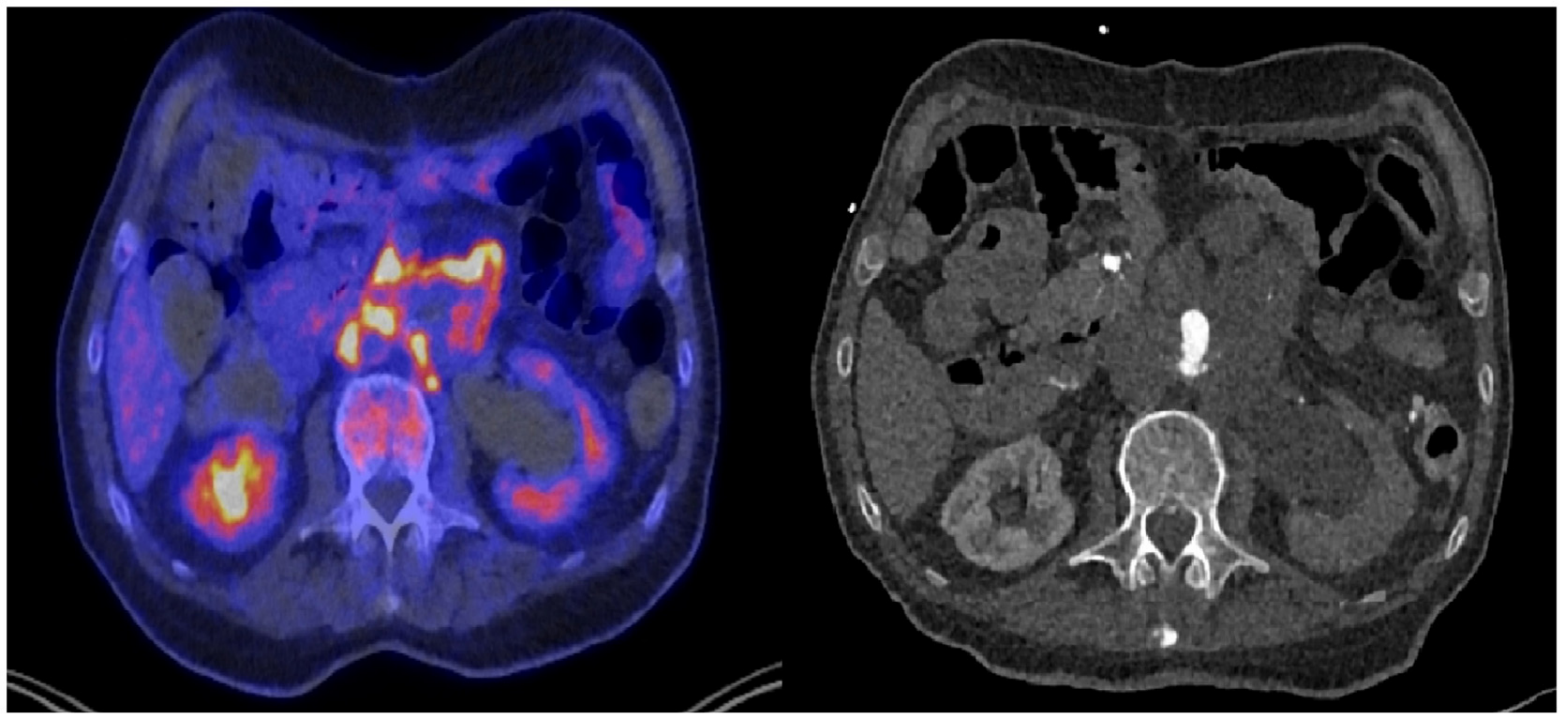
Positron emission tomography (PET)-computed tomography (CT) scan showing ^18^fluorodeoxyglucose (FDG) uptake around the venous reconstruction of the abdominal aorta.
